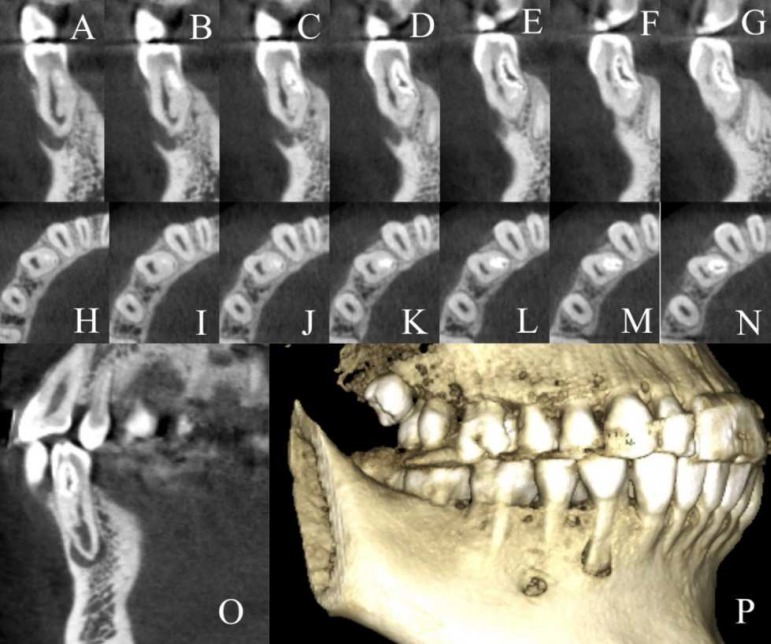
A to O) Confirmation of the extent of dens invaginatus and the size of periapical pathosis using cone-beam computed tomography images; P) Cone-beam computed tomography images ‎3D reconstruction (Buccal cortex perforation was observed adjacent to the affected tooth
